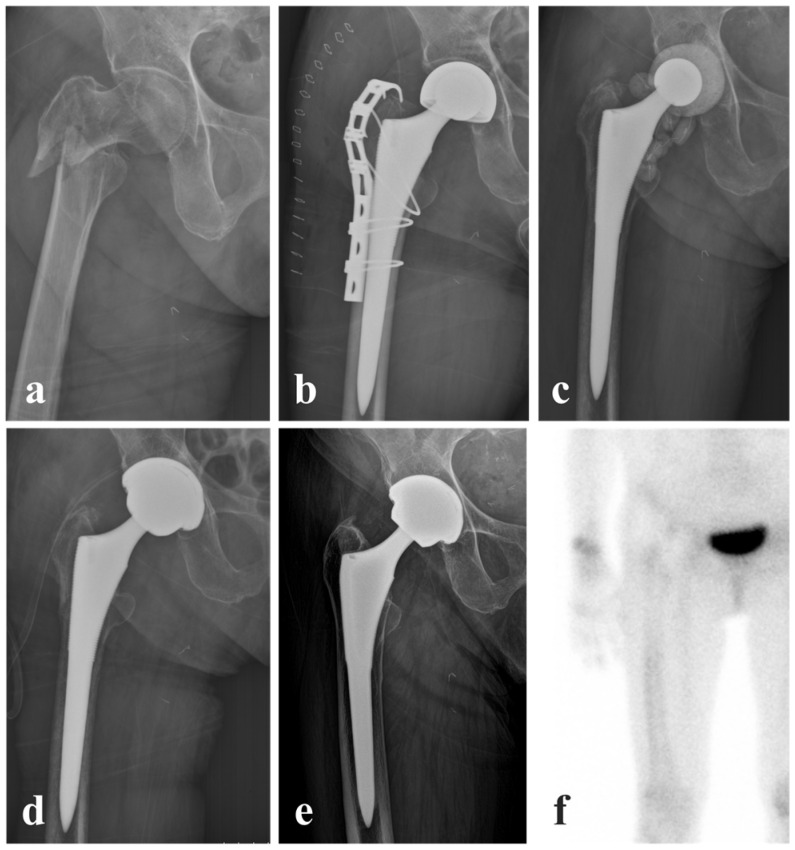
A case of a 74-year-old female treated with complete two-stage revision with femoral stem retention. (a) Preoperative radiograph showing an intertrochanteric fracture. (b) Postoperative radiograph after index arthroplasty with an additional trochanteric reattachment device. (c) Postoperative radiograph after the first-stage revision with femoral stem retention. (d) Immediate postoperative radiograph after the second-stage revision with definitive THA. (e) Follow-up radiograph obtained 13.3 years postoperatively. (f) Bone scan image obtained 10 years after surgery, showing negative uptake around the right hip joint.
